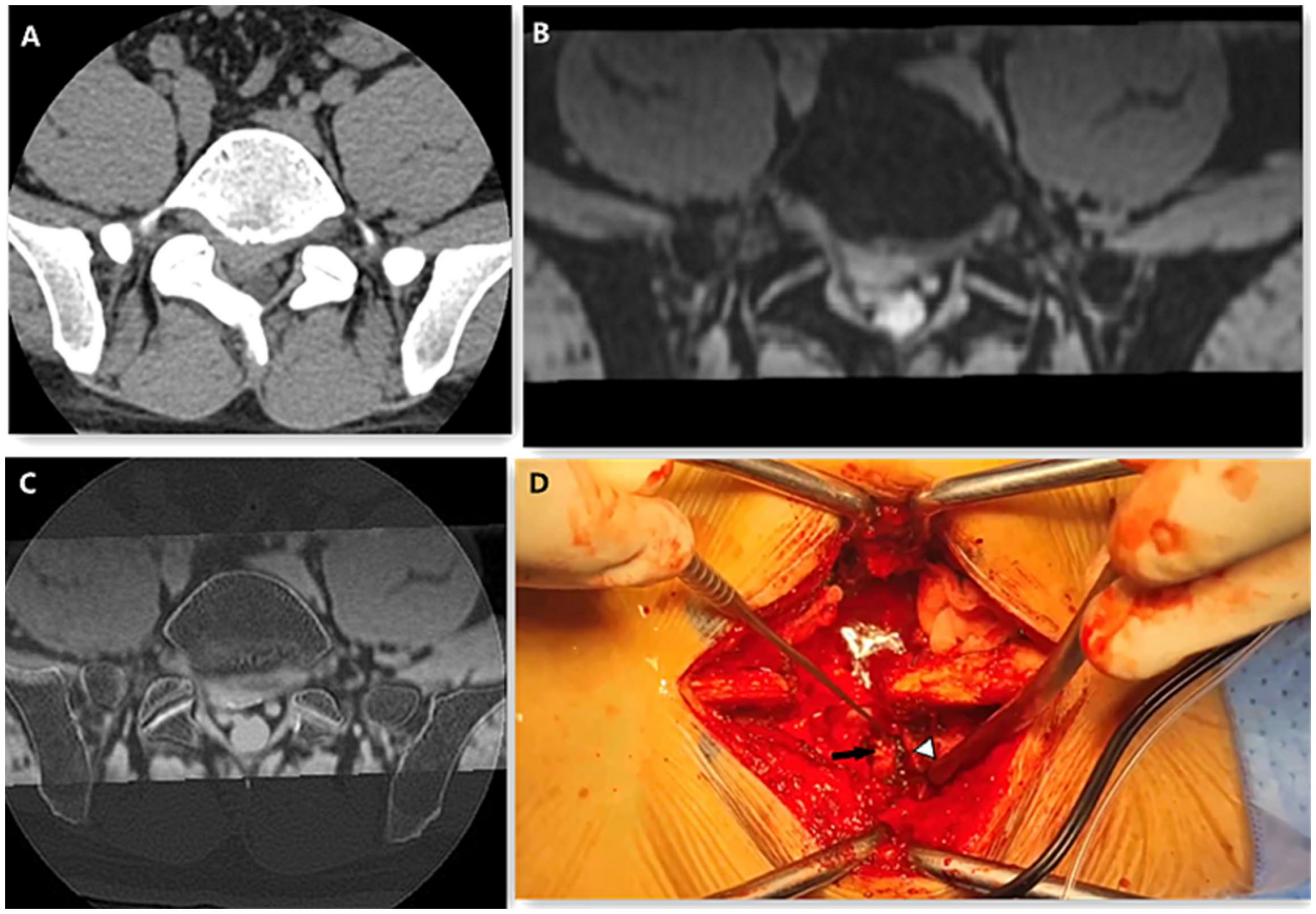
A 41-year-old male presented with L5/S1 disc herniation and right nerve root compression. The CT axial (A) image scores of bony structure, intervertebral disc and nerve root were 5, 4, 3. The scores of 3D MERGE (B) of bony structure, intervertebral disc and nerve root were 3, 5, 5. The image quality score of CT-3D MERGE fusion image (C) were 5, 5, 5. On the Photo of posterior lumbar interbody fusion surgery (D), the black arrow shows the herniated disc and the white arrow head shows the nerve root.
